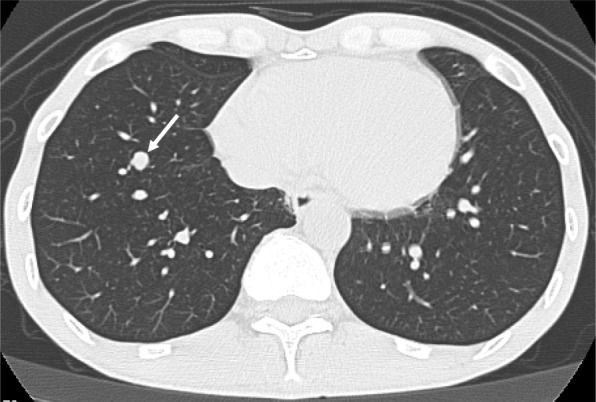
Computed tomography showed an enlarged nodule in the right lower lung lobe (arrow)
